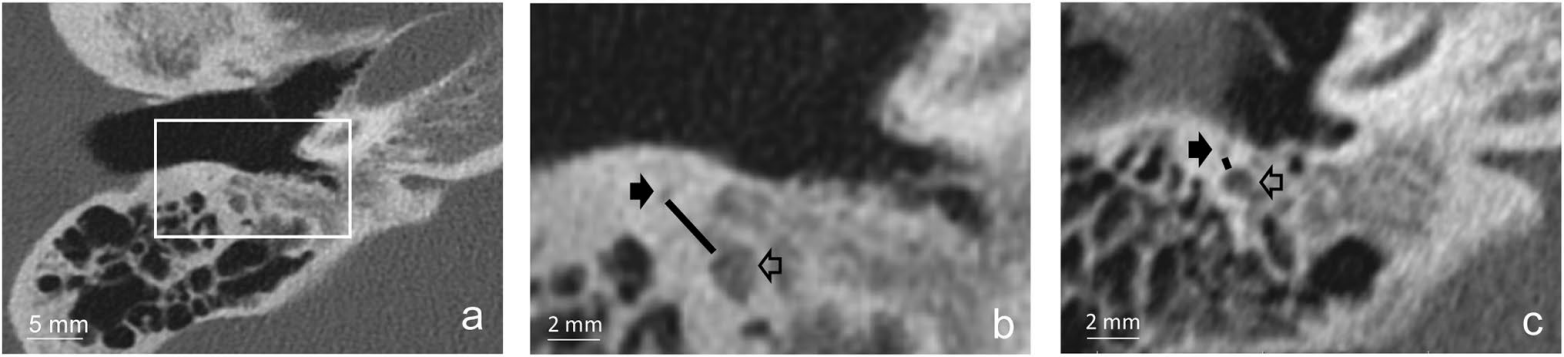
a Overview of the preoperative axial high resolution CT scan of the right temporal bone. b Magnification (× 2.5) of the same axial high resolution CT scan of the right temporal bone. Black arrow depicts the chorda tympani nerve (CTN), and the unfilled arrow the facial nerve (FN). The line between these two nerves is the FN-CTN distance. This case had a FN-CTN distance of 2.9 mm. c Axial high resolution CT scan of the right temporal bone of another patient. This case had a small FN-CTN distance of 0.6 mm
